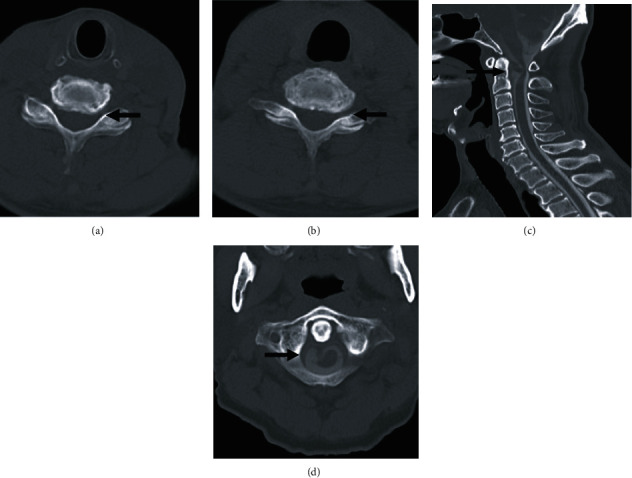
Noncontrast-enhanced computed tomography (CT) and CT myelography. Foraminal stenosis of the left C6-7 intervertebral foramen was identified in the horizontal section ((a, b), arrow). CT myelography showed an intradural extramedullary cyst ((c) sagittal; (d) horizontal, arrow).
